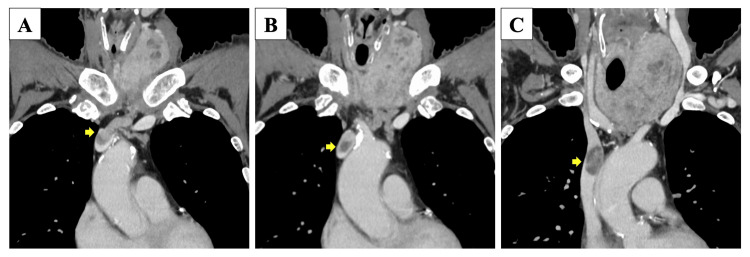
(A-C) Contrast-enhanced CT showing the thyroid tumor extending through the inferior thyroid vein, left brachiocephalic vein, and into the superior vena cava (SVC), suggesting tumor extension into the SVC (arrow).
